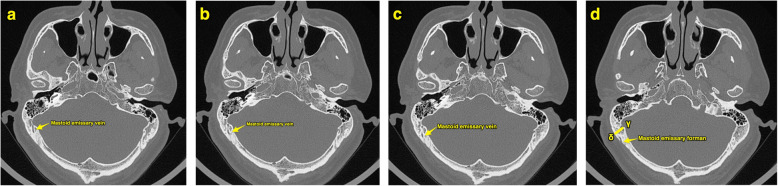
The course of mastoid emissary vein and slice B. The course of mastoid emissary vein can be traced through the continuous CT images (a–c). The slice B (d) should reveal the mastoid emissary foramen and the locations of point γ and point δ. The distance between point δ and the mastoid emissary foramen should be measured
